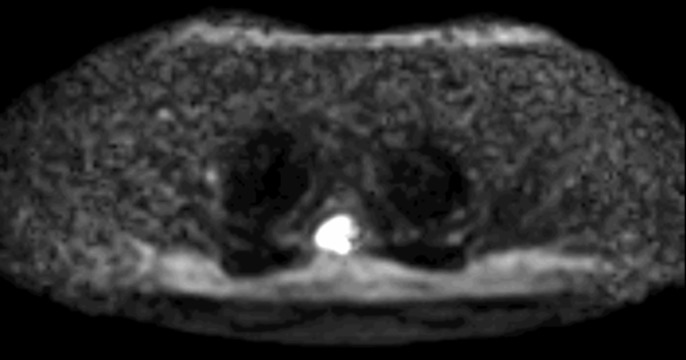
Preoperative DWI MRI Preoperative axial DWI MRI of the T3 thoracic lesion demonstrating marked diffusion restriction.
